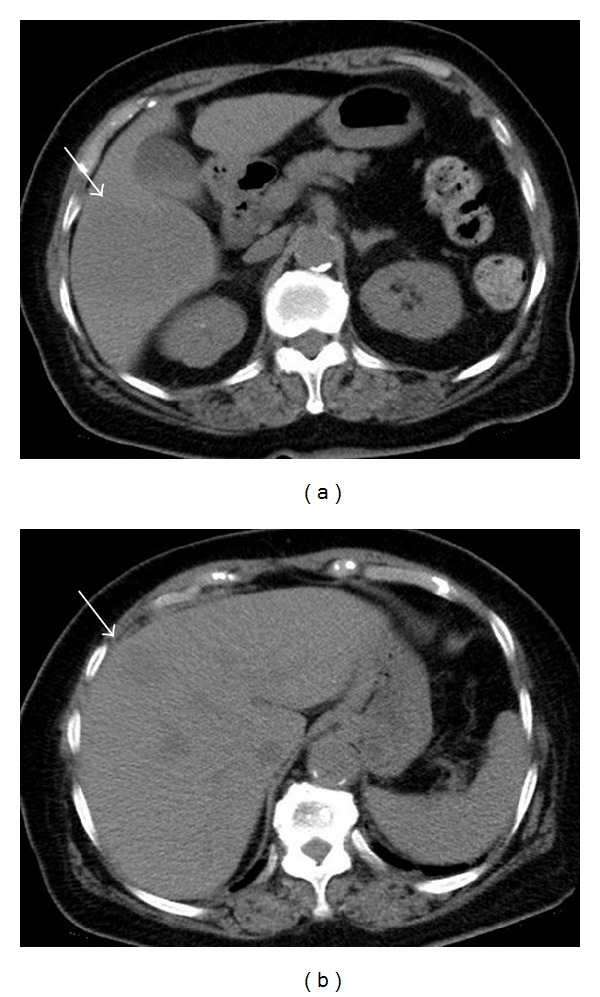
Noncontrast abdominal computed tomography. Low-density masses were revealed at segments 5 and 8, suggestive of a liver abscess ((a) and (b)).
